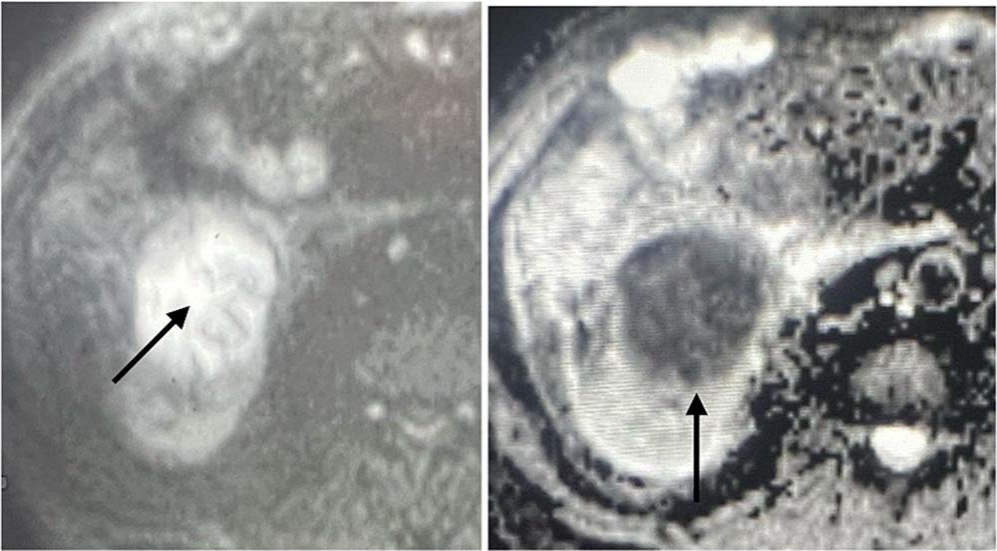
DWI- ADC map shows strong internal restricted diffusion which can present in both abscess as well as tumor (arrow).
